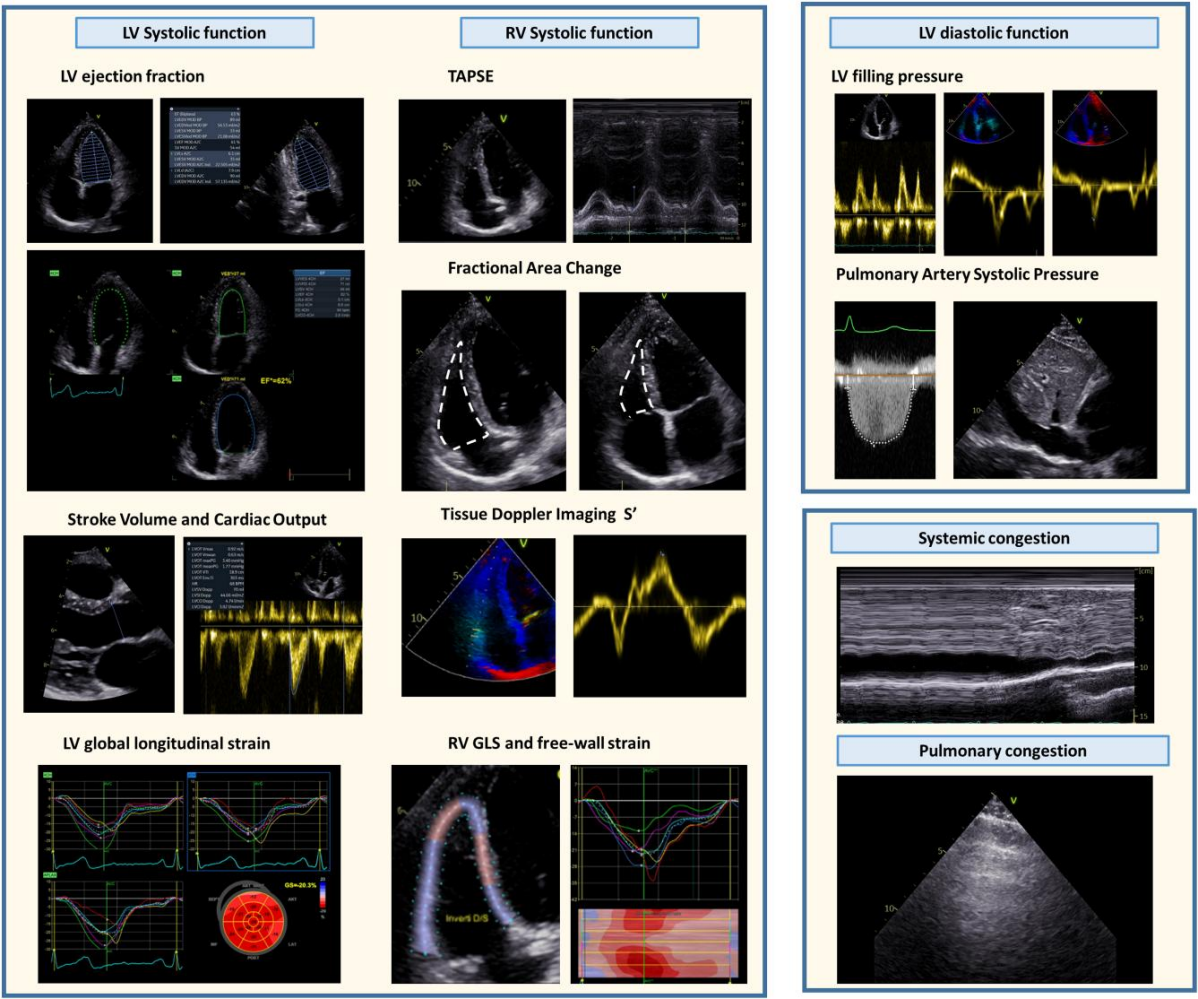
Most common conventional and advanced echocardiography-derived haemodynamic parameters. GLS: global longitudinal strain; LV: left ventricular; RV: right ventricular; TAPSE: tricuspid annular plane systolic excursion.
